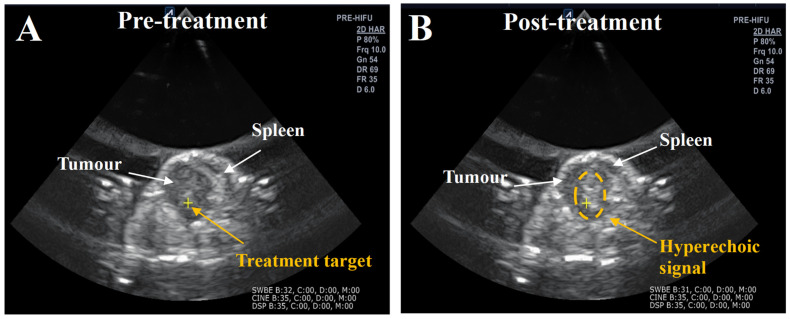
Examples of ultrasound images of BH-treated KPC tumours immediately before and after treatment. Ultrasound images of subject 3 (Table 1-combination group) before (A) and after (B) the BH treatment. Before treatment, the tumour has a round shape, is less echogenic than its surroundings, and can be readily distinguished from the spleen. The yellow cross denotes a treatment target position. Immediately after treatment, a bright hyperechoic signal is typically seen within the tumour, indicated by the amber circle and arrow, which acts as an independent indicator for the induction of acoustic cavitation in the tumour.
